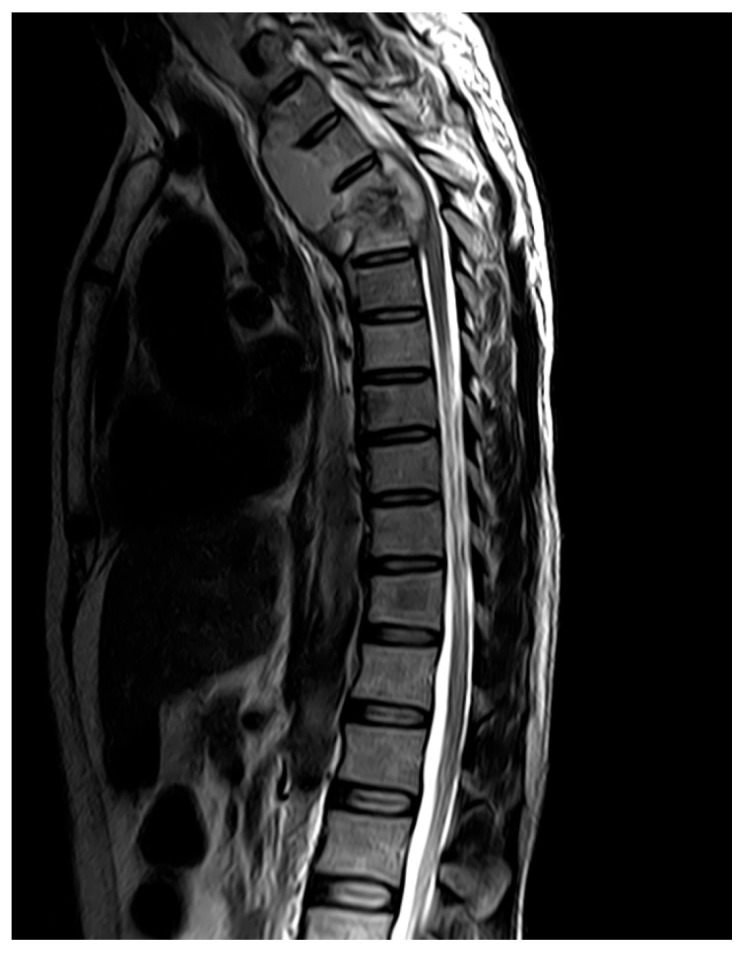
T2-weighted MRI of the thoracic spine in a patient with tuberculous spondylitis showing destruction of the T4 and T5 vertebral bodies and associated prevertebral and paravertebral abscess formation. Case courtesy of Pir Abdul Ahad Aziz Qureshi, Radiopaedia.org, rID: 73438, licensed under CC-NC-BY-SA 3.0 [38].
